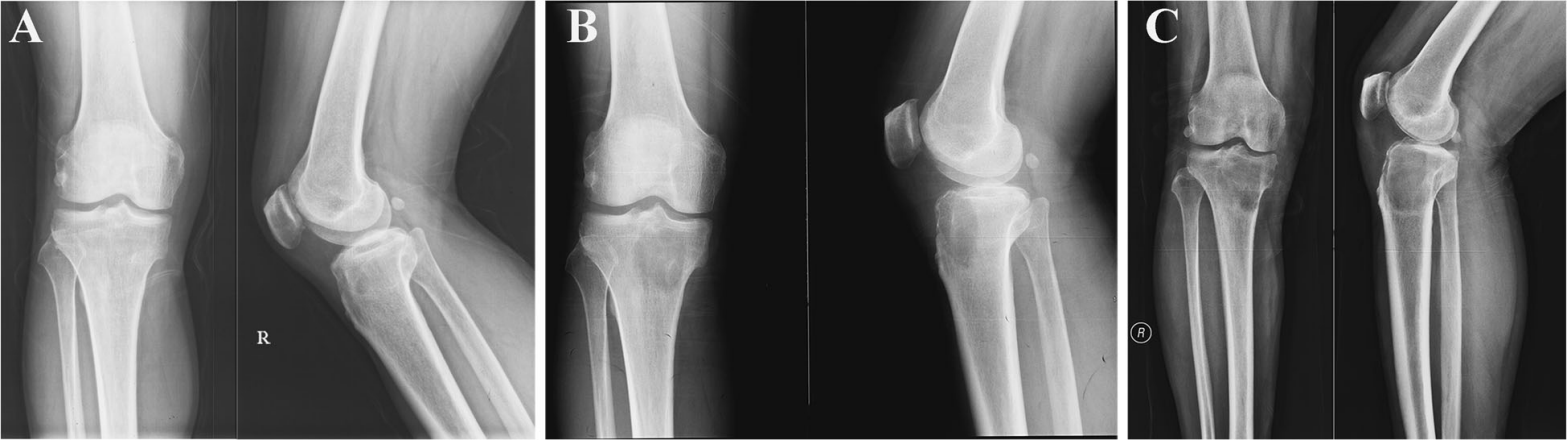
a Anteroposterior radiograph of the right knee showed a small lytic lesion in the proximal tibia with a diameter of 1 cm. b After five months, the lesion extended, and has a non-sclerotic margin medially (arrowheads). c After six months, the lesion shows typical appearance of GCTB which extends to the subchondral bone that is eccentric in location and extends to the subchondral bone with a sclerotic margin
